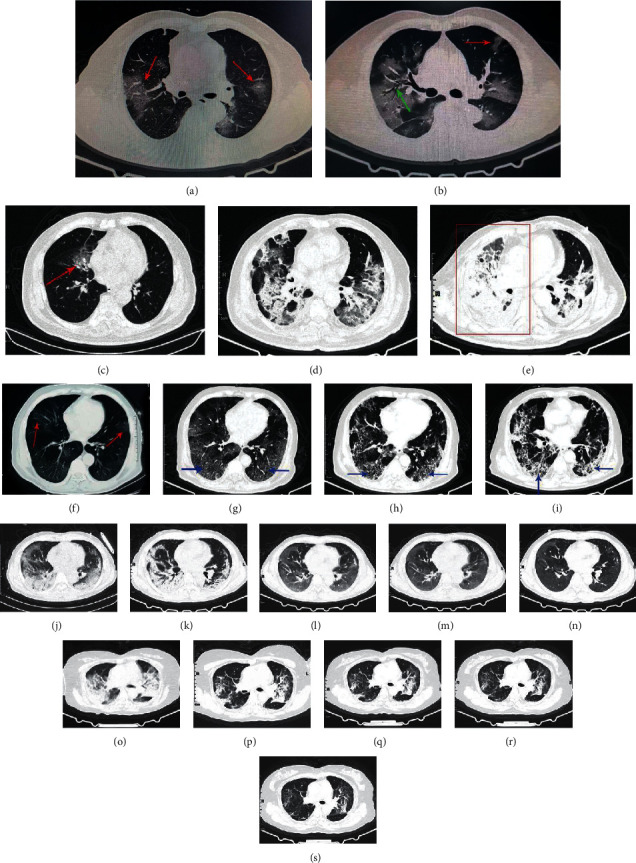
Chest computed tomographic images of COVID-19 patients. (a) and (b) show typical ground-glass opacities (marked by red arrow) and patchy shadows in both lungs of COVID-19 patients. The green arrow shows bronchial inflation sign. Case 1: (c), (d), and (e) are chest CT images of a 75-year-old dead man at the time of onset, the 14th day, and the 21st day after onset, respectively. The patient's lung lesions became more and more serious, and the right lung finally presented a “white lung” like change (as shown in red rectangle). Case 2: (f), (g), (h), and (i) are chest CT images of a 69-year-old severe male patient on admission, the 7th, 14th , and 21st days, respectively. The lesions showed fibrotic progression. The part pointed by the blue arrow shows “paving stone sign.” Case 3: (j), (k), (l), (m), and (n) are chest CT images of a 60-year-old severe male patient on admission, the 6th, 12th, 18th, and 24th days, respectively. The patient's lung lesions gradually improved after comprehensive treatment. Case 4: (o), (p), (q), (r), and (s) are chest CT images of a 58-year-old woman on admission, the 6th, 12th, 18th, and 26th days, respectively. The patient's bilateral lung lesions gradually improved after symptomatic treatment.
